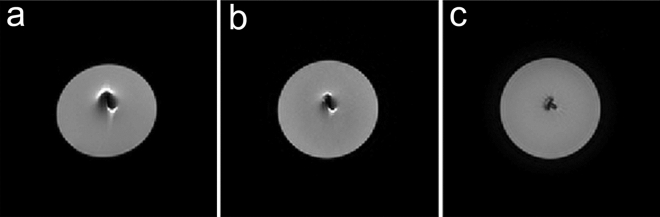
Images of an aneurysm clip embedded in agarose gel obtained by three DWI sequences. Image distortion around the clip is the largest in SS-EPI DWI (a), second largest in RESOLVE DWI (b), and the minimal in TGSE-BLADE DWI (c). Note the distorted contour of agarose gel in SS-EPI DWI and RESOLVE DWI.
